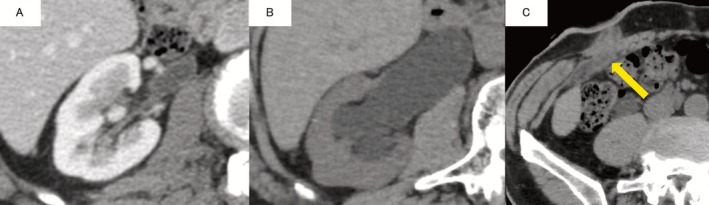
Postoperative CT findings. The patient had mild hydronephrosis after surgery (A) but this had progressed 3 years later (B). In addition, subcutaneous wall thickening and periureteral involvement were observed near the opening of the right ureter (C).
